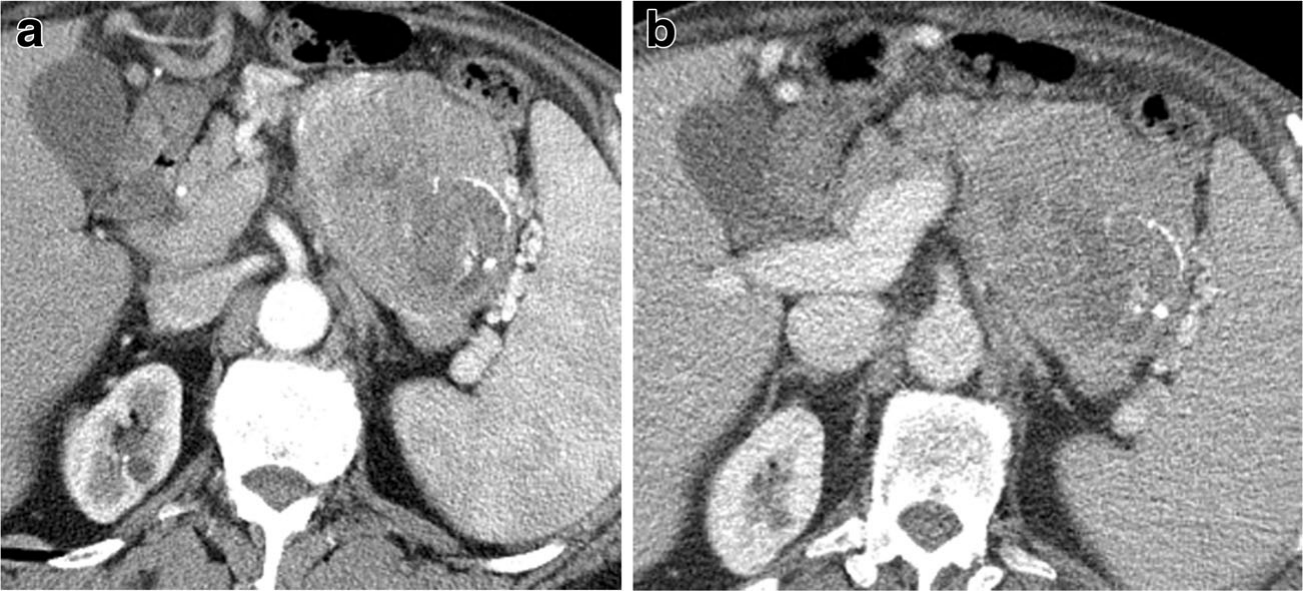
Non-functioning NEN. CT examination: huge inhomogeneously hypovascular pancreatic body-tail mass with necrotic areas and intralesional calcifications studied in the pancreatic (a) and venous (b) phases
